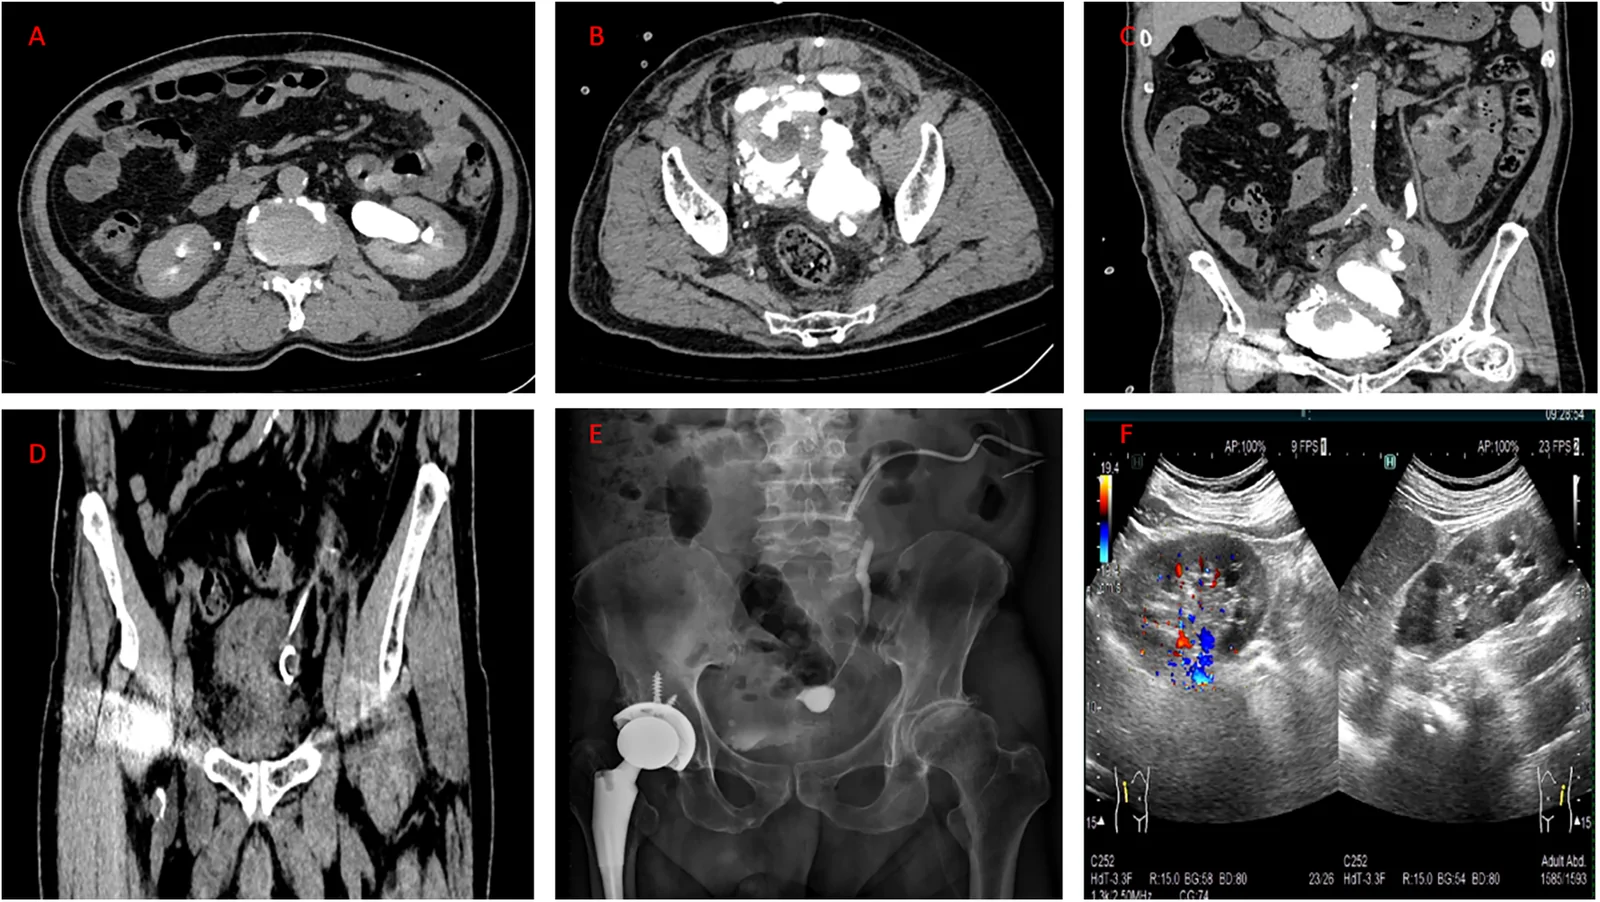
Imaging findings before surgery and during postoperative follow-up. (A) Preoperative axial computed tomography urography (CTU) urography image showing dilation and hydronephrosis of the left renal pelvis and proximal ureter. (B) Preoperative axial pelvic CTU urography image showing contrast extravasation around the operative site. (C) Preoperative coronal CTU urography reconstruction showing left ureteral dilation with contrast extravasation at the operative site. (D) Postoperative coronal lower abdominal and pelvic CT image showing the distal end of the double-J stent within the bladder. (E) Antegrade urography performed 6 weeks after surgery showing a patent left ureteral anastomosis to the bladder diverticulum, with contrast passage into the bladder lumen and no obvious leakage. (F) Urinary ultrasonography performed 4 months after surgery showing no obvious hydronephrosis of the left kidney or left ureter.
